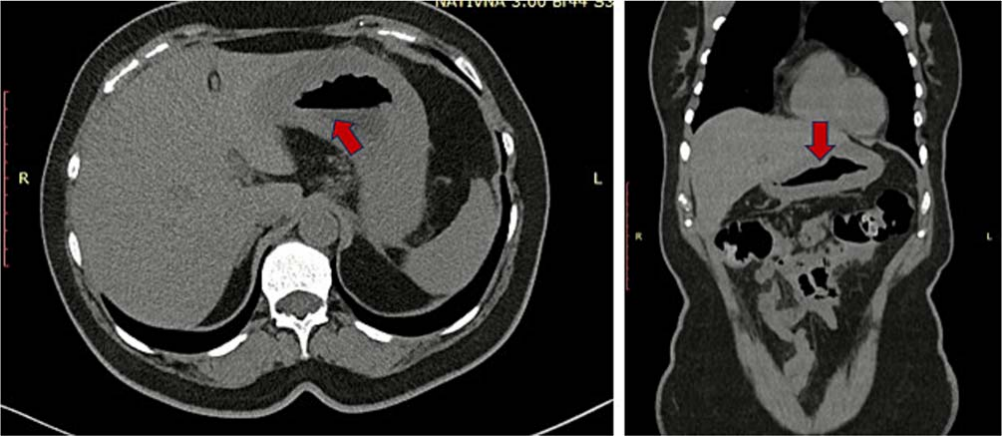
CT scan before surgery (red arrow indicating thick gastric wall).
